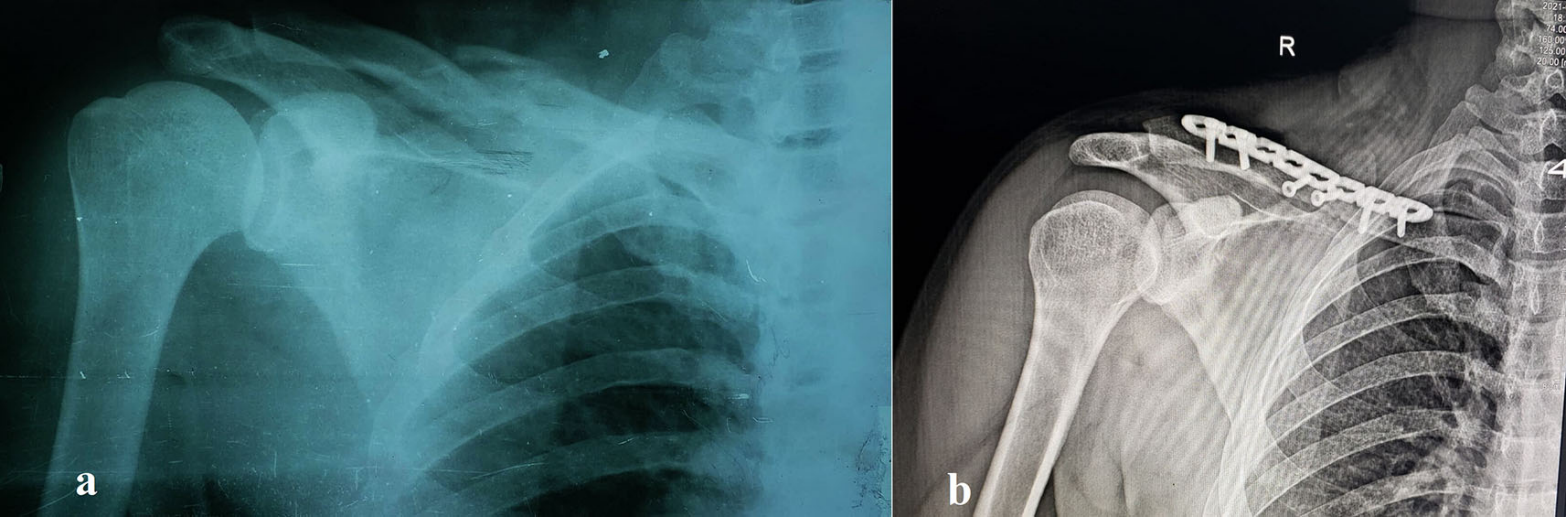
Pre- and postoperative radiographs of a 26-year-old female with comminuted midshaft clavicular fracture. (a) Preoperative anteroposterior radiograph showing a displaced, severely comminuted midshaft fracture of the right clavicle in a 26-year-old female patient. (b) Postoperative anteroposterior radiograph of the same patient after open reduction and internal fixation (ORIF) with a precontoured superior locking plate (Orthomed E, 8-hole, titanium). Note the anatomical reduction of the fracture and the use of locking screws that engage at least four cortices on either side of the fracture. Two lag screws were used to stabilize the butterfly fragments.
